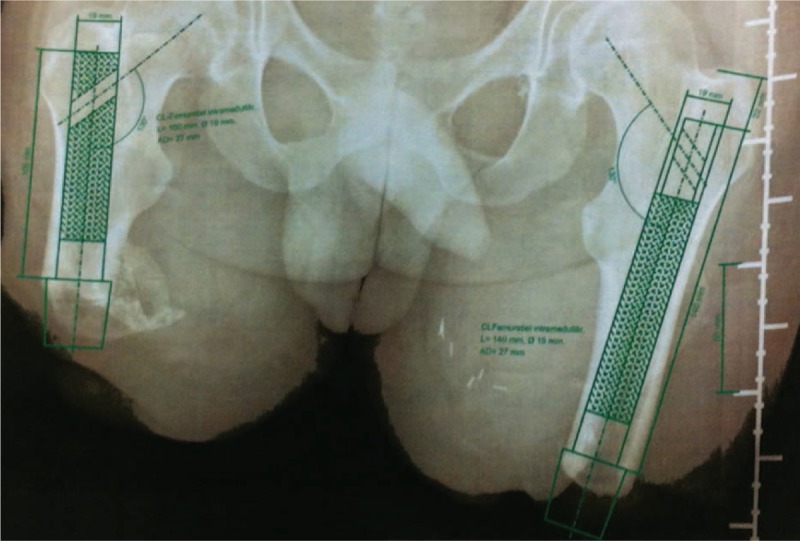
X-ray of participant stumps with computerized preparation for the osseointergration procedure.
